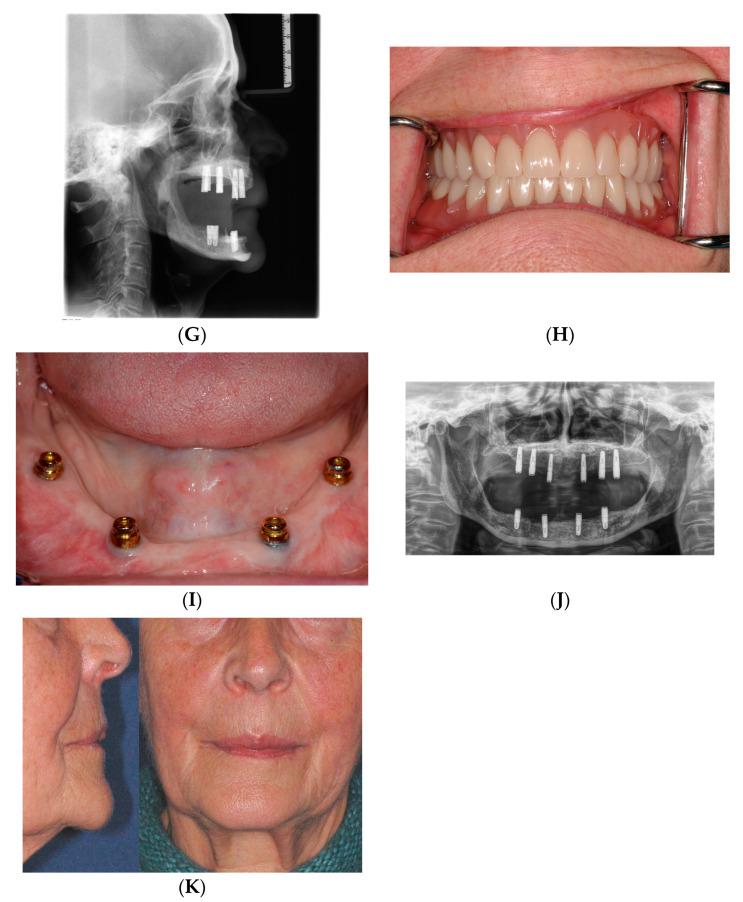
Vertical bone augmentation after severe alveolar atrophy in an edentulous 70-year-old female patient. (A) Severely atrophic maxilla and mandible (8 mm symphysis height). (B) Almost no fixed gingiva left; the floor of the mouth stands above the alveolar crest. (C) Intraoperative view. Sandwich osteotomy, space filled with mixed bone substitute. (D) Intraoperative view. Le Fort 1 interpositional grafting in the maxilla, supplemented with small strips of iliac block bone grafts. (E) Postoperative lateral cephalogram, vertical bone gain in maxilla and mandible. (F) Postoperative panoramic X-ray shows the utilization of the new bone for dental implants of regular length. (G) Lateral cephalogram, parallel positioning of the dental implants, no flaring of the implant axes in the maxilla. (H) Prosthetic treatment with full dentures on locator abutments. (I) Spontaneous re-appearance of the fixed gingival tissue without soft tissue augmentation of a vestibuloplasty (compare (B)). (J) Ten years later, almost no loss or resorption of the augmented bone in both jaws, the bone-protective effect of the dental implants. (K) Facial aesthetics: After vertical bone augmentation and prosthetic treatment, reduction of wrinkles and prominence of the vermilions, no drooling, adequate lower face height.
